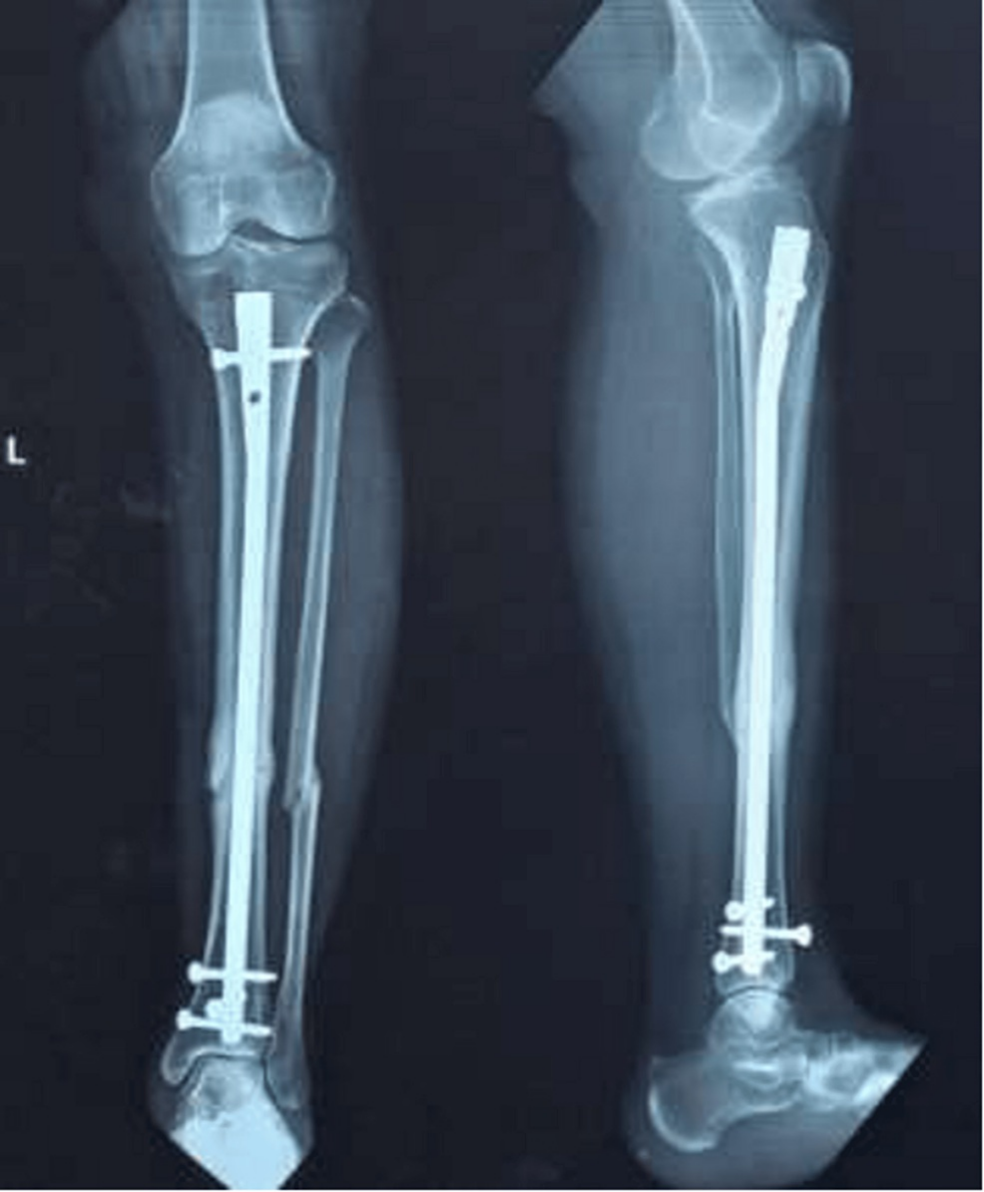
One-month follow-up radiograph
